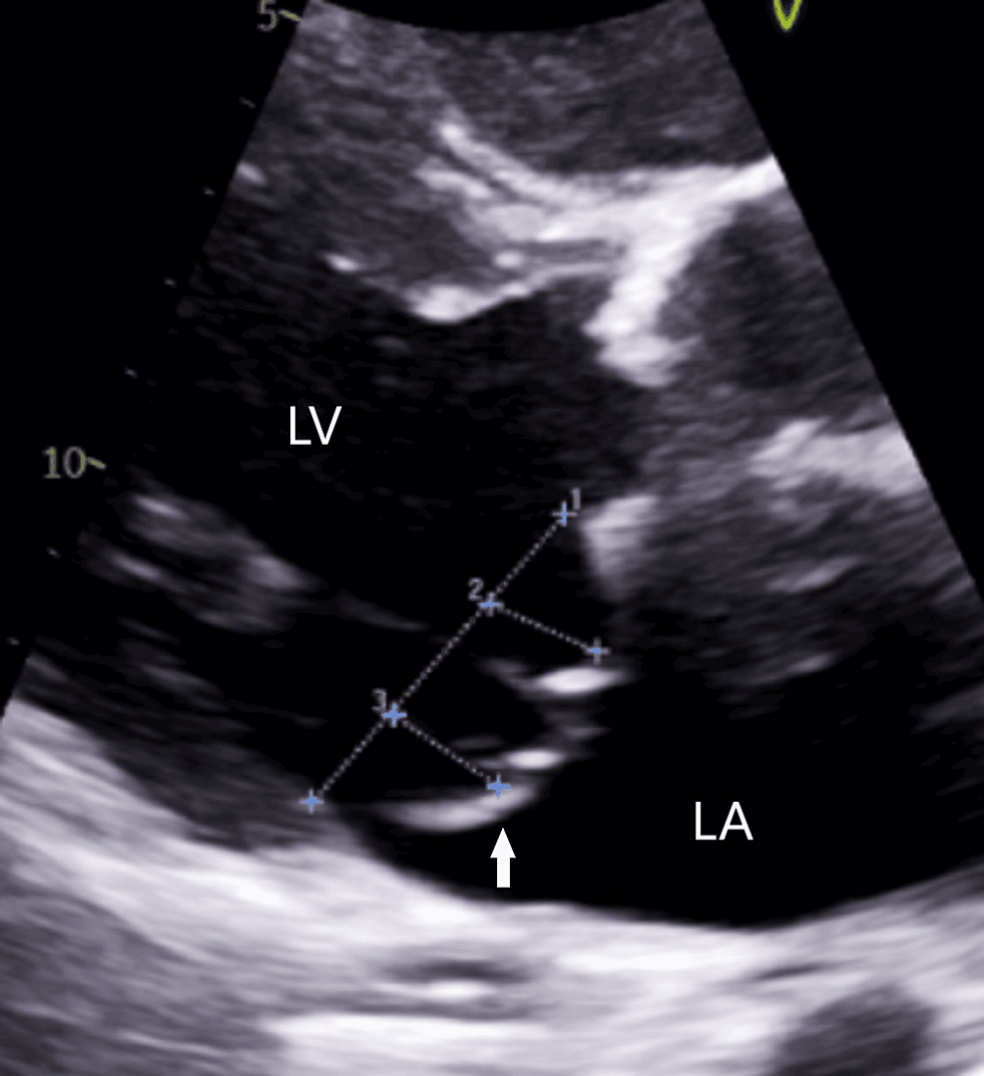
Transthoracic echocardiogram parasternal view showing mitral valve prolapse (arrow). The mitral valve opening was 3.9 cm (1). The mitral valve prolapse was 1.2 cm (2) and 1.3 cm (3) LA: Left Atrium; LV: Left Ventricle
